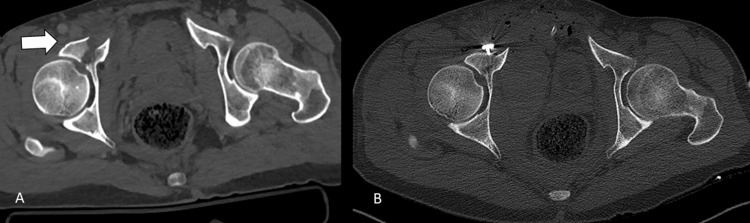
Computed tomography sections A) preoperative, B) postoperative
